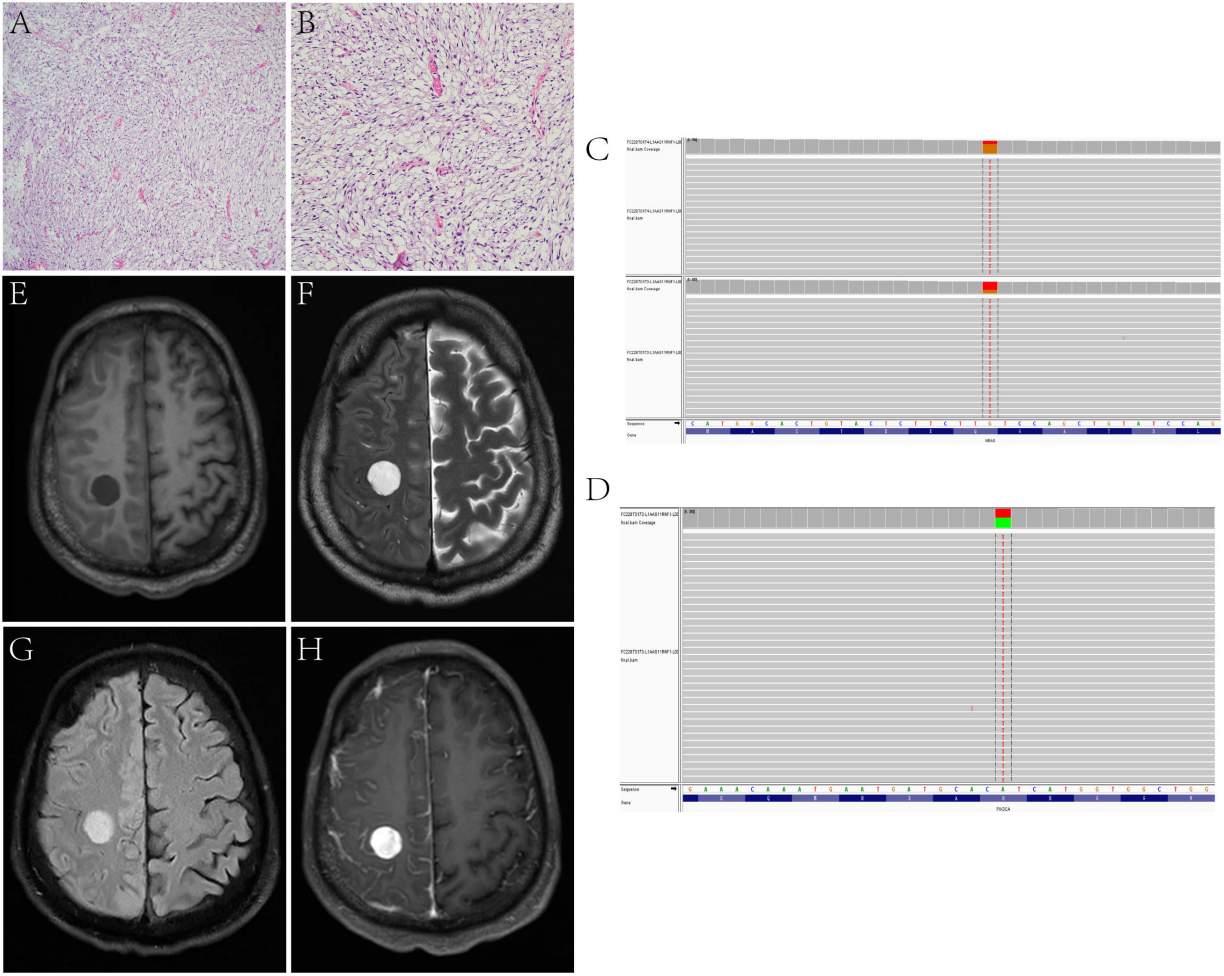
In Case 3, the tumor cells are fusiform, moderately dense, with obviously myxoid stroma and lengthened, curvilinear, thin-walled blood vessels (A, B). Target NGS sequencing finds the mutation of NRAS (C) and PIK3CA (D). MRI reveals that a sub-round solid lesion is located in the right frontal lobe, T1-weight imaging (T1WI) (E) shows hypointensity, T2-weight imaging (T2WI) (F) and T2 Fluid Attenuated Inversion Recovery (T2 FLAIR) (G) shows hyperintensity, and contrast-enhanced T1WI (H) shows inhomogeneous enhancement.
